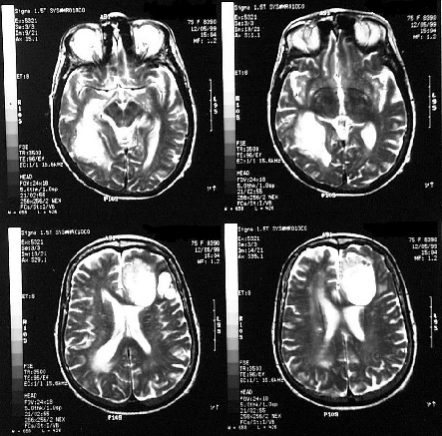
Magnetic resonance imaging (MRI) of the brain obtained after first drainage.
